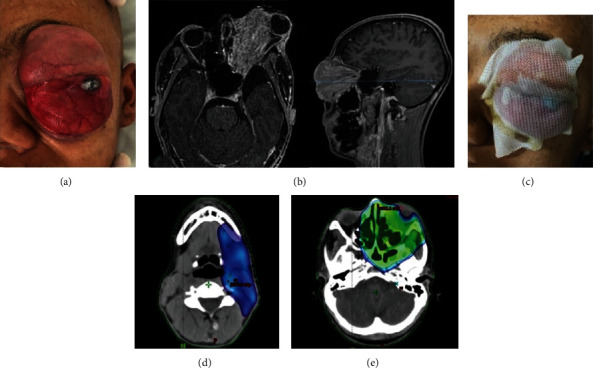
Clinical examination after neoadjuvant QT. (a) Partial response to treatment was observed with increased tumor volume. (b) MRI in axial and sagittal slices confirmed. (c) CT for RT planning. (d) PTV contouring included submental lymph nodes. (e) CTV for tumor irradiation.
